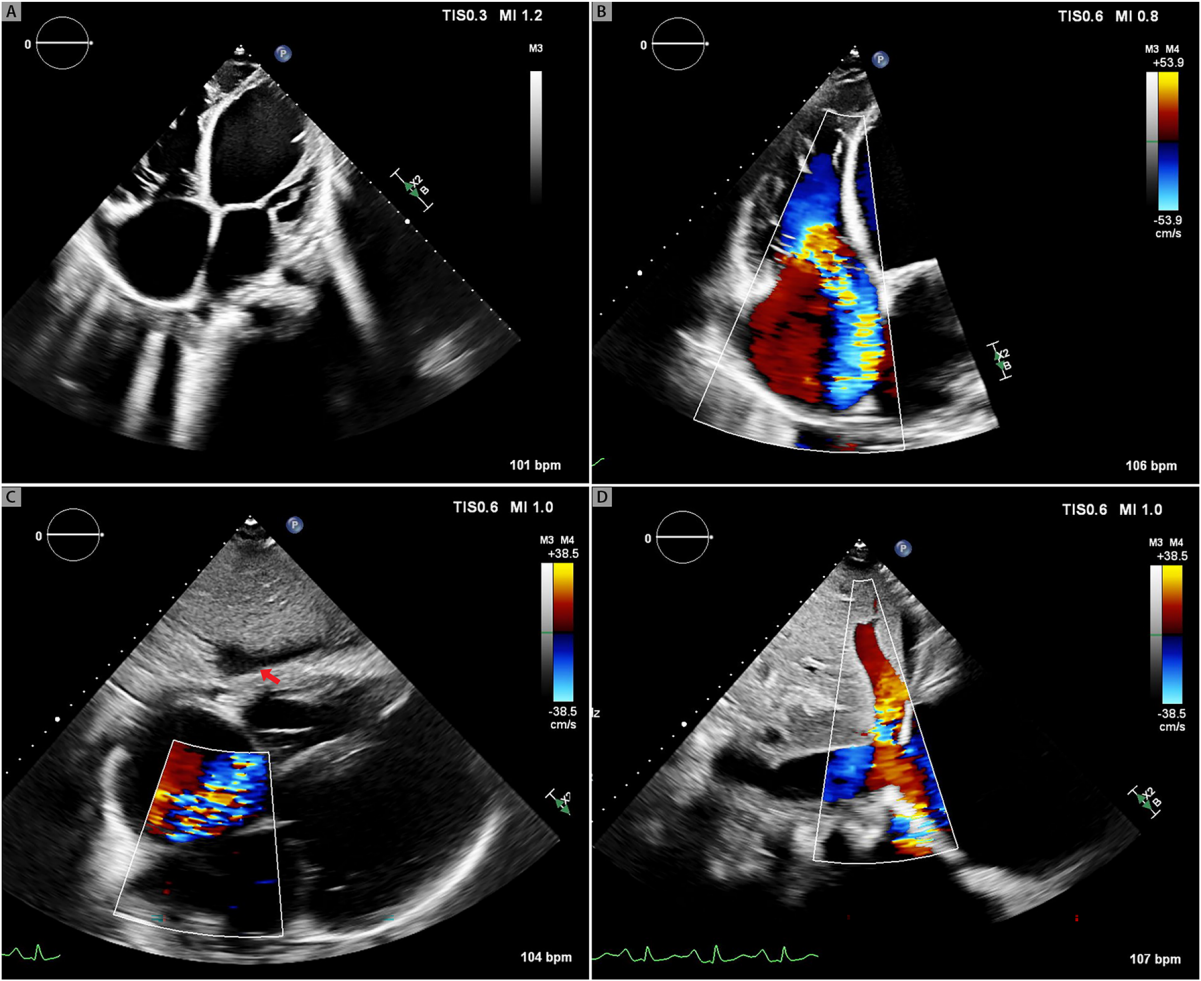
Echocardiogram of severe heart failure and severe tricuspid regurgitation. 2D image showing four-chamber enlargement and severe biventricular dysfunction. (Panel A). Severe tricuspid regurgitation (panel B). Perihepatic fluid (red arrow, panel C). Systolic flow reversal in the hepatic vein by color Doppler (panel D).
